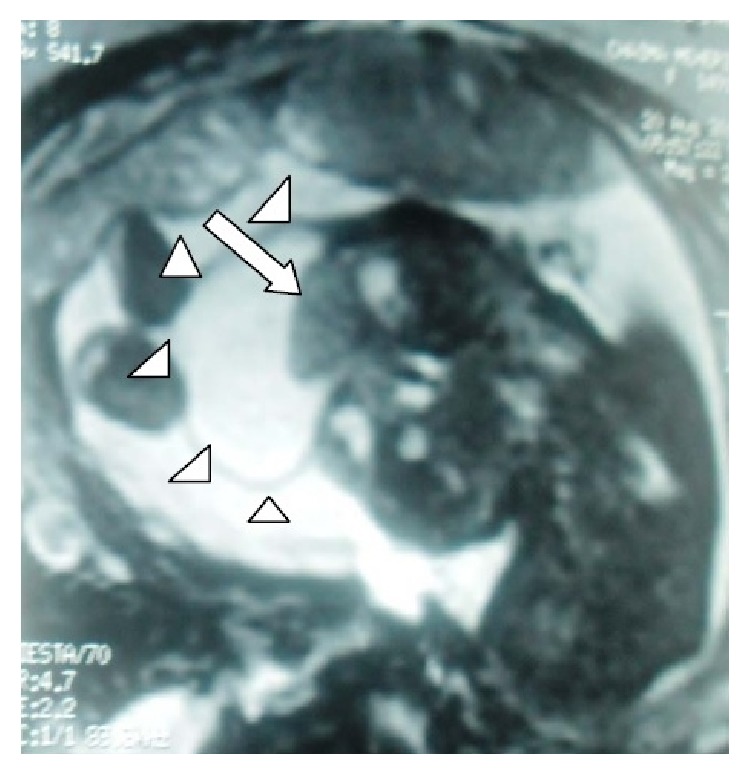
Prenatal MRI showing a small round midline lesion with herniation of brain parenchyma (arrow).
